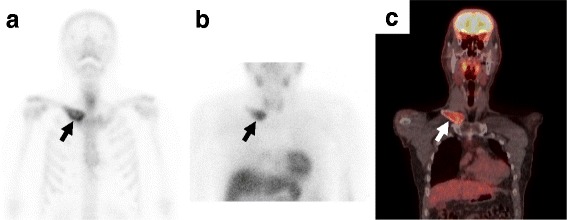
Radionuclear medicine. (a) Bone scintigraphy showed marked uptake in the proximal clavicle, and (b) thallium-201 scintigraphy showed strong accumulation (normal/tumor ratio, 2.31), suggesting an abundant blood supply to the tumor. (c) Positron-emission tomography demonstrated strong accumulation of fluorine-18-2-fluoro-2-deoxy-d-glucose (SUVmax, 6.0) in the proximal part of the right clavicle, but there were no primary tumors or metastases in other body sites
